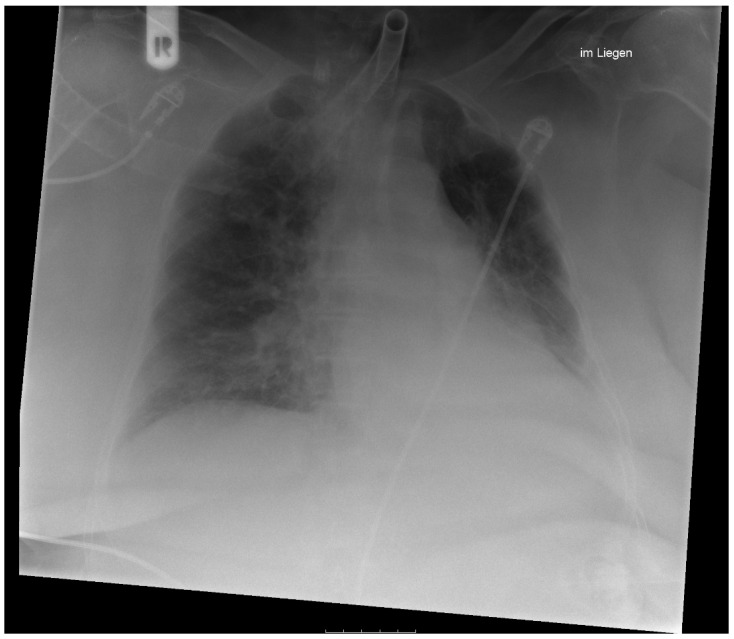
Chest X-ray from the fourth postoperative day: Twisted image with lateralization of the mediastinum to the left. Inserted tracheal cannula, the tip of which projects approximately 6.5 cm cranially to the carina onto the tracheal lightening band. The left dome of the diaphragm is not sharply delineated. Marked areal compression left-retrocardially. Prominent pulmonary hili on both sides as well as somewhat blurred vascular markings. In the supine position, no evidence of a pneumothorax. Assessment: Compaction retrocardially on the left side, consistent with a pneumonic infiltrate. Mild PV congestion.
